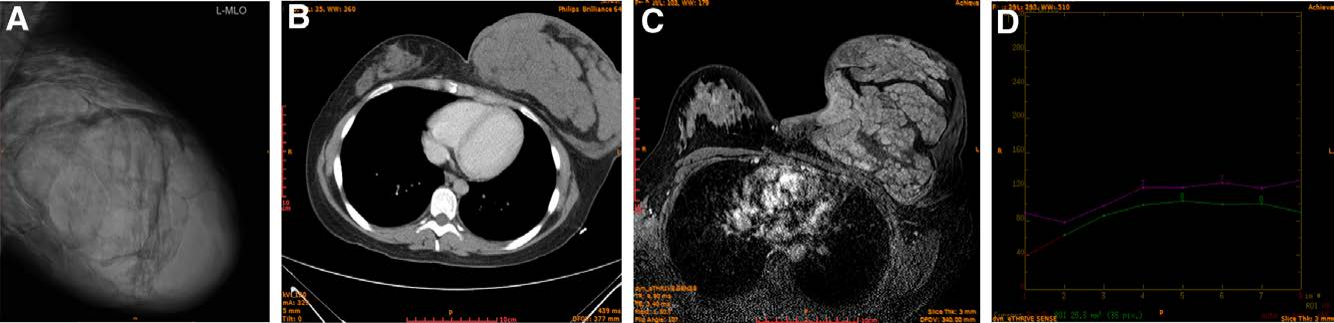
Preoperative imaging data of breasts. Mediolateral oblique mammogram (A). Computed tomography (CT) scan (B). Magnetic resonance imaging (MRI) scan (C).
